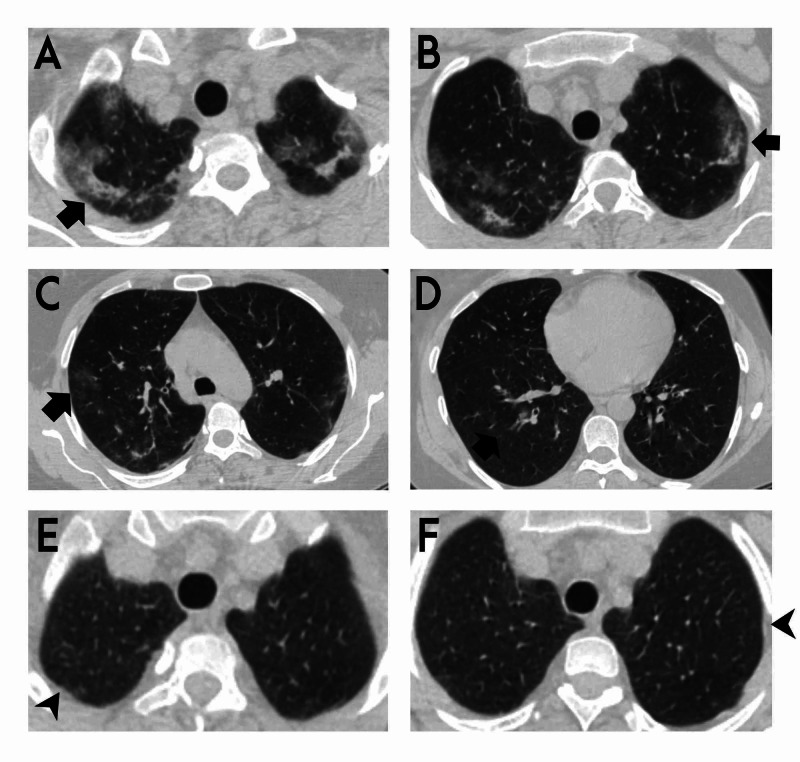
Thorax CT sections of the patient (A-D) Bilateral ground-glass opacities with peripheral and upper zone predominance, as shown on thorax CT by arrows. (E and F) After four weeks of treatment with oral prednisone, lesions disappeared in control thorax CT, as shown by arrowheads.
